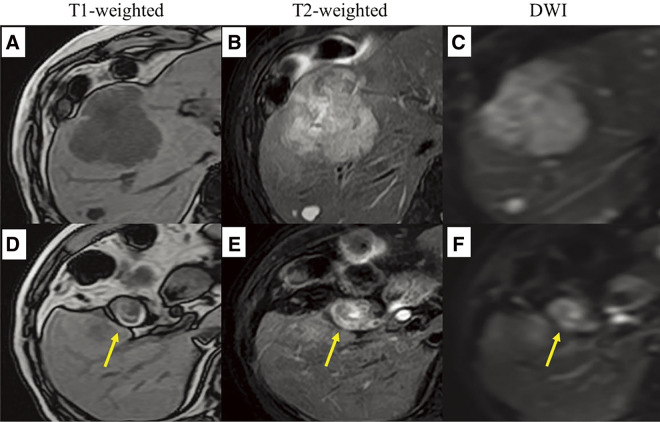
The liver lesion showed low intensity on T1-weighted images, high intensity on T2-weighted images, and diffusion restriction on DWI (A–C). The gallbladder lesion showed similar findings (D–F: arrow). DWI, diffusion-weighted image
